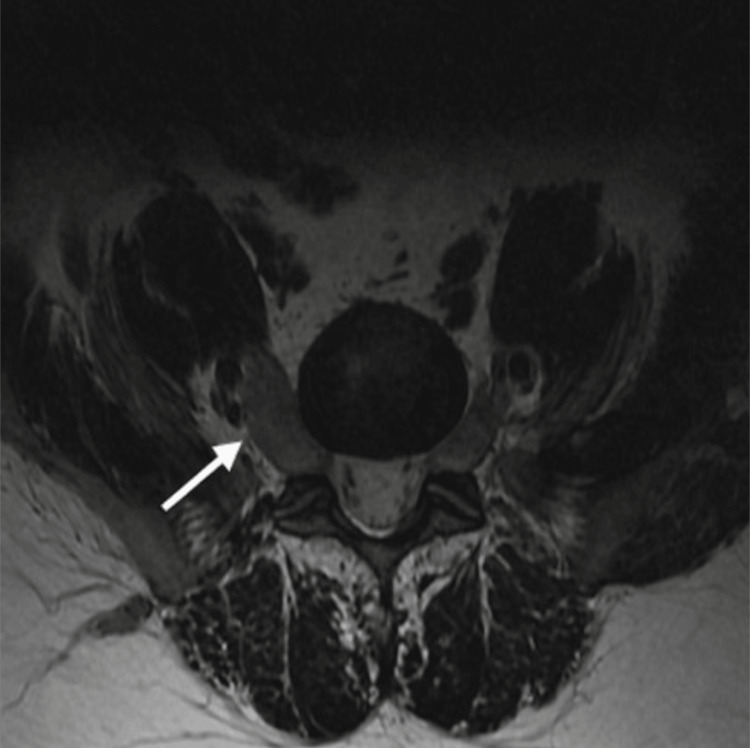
Axial T2-weighted MRI of the lumbar spine Axial MRI demonstrating diffuse symmetric enlargement of the lumbosacral nerve roots without abnormal enhancement. The hypertrophic nerve roots (white arrow) produce a clustered appearance within the intervertebral foramen.
